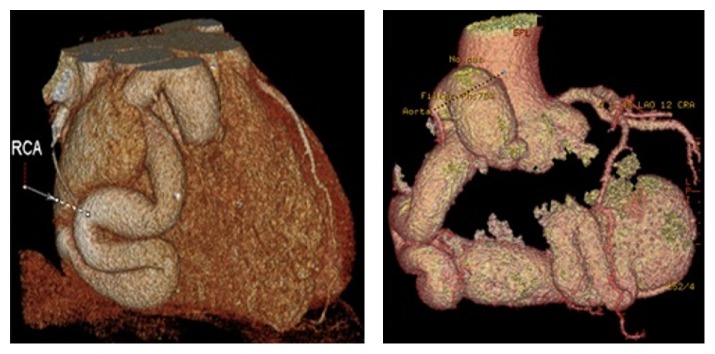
Three-dimensional reconstructions of CT images demonstrating the course of the aneurysmal right coronary artery on the surface of the heart.
